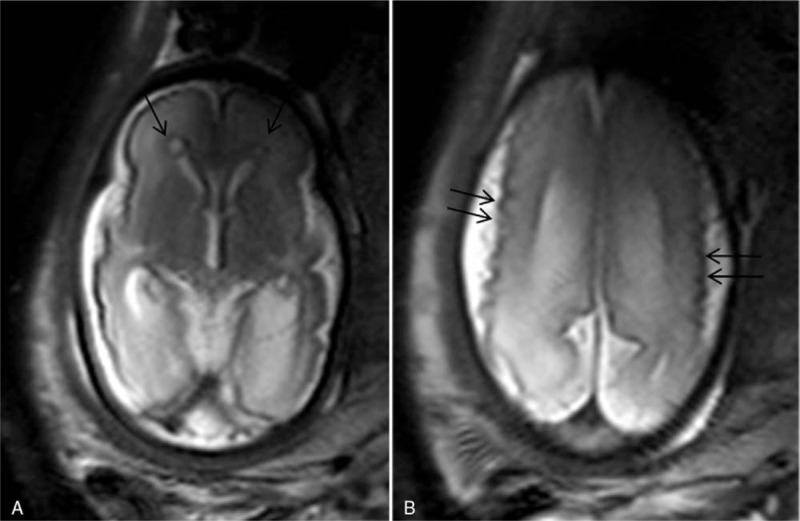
A 33 weeks gestation patient was referred for magnetic resonance imaging (MRI) because a positive cytomegalovirus serology at the first pregnancy trimester, and cerebral fetal lesions viewed by sonography (not shown). Axial T2 turbo-spin echo (TSE) single-shot images shows microcephaly, micropolygyria (double arrows), and periventricular cavities (arrows) (A and B).
